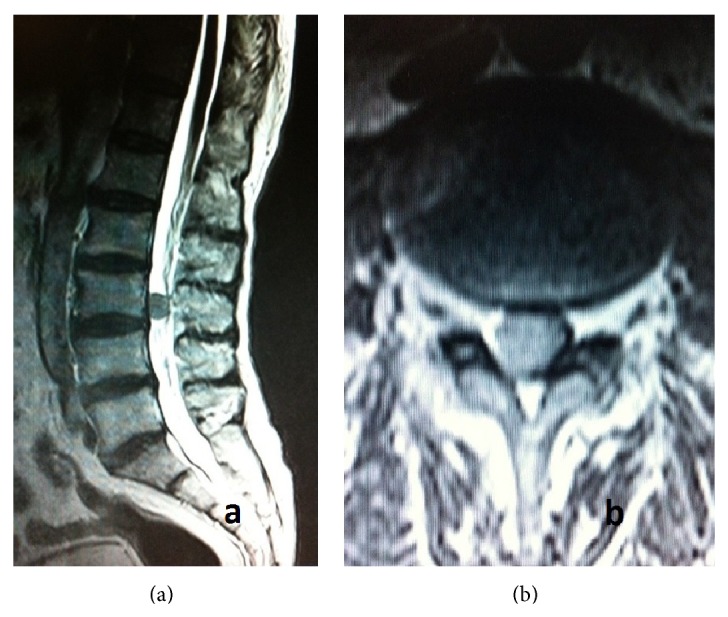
(a) Preoperative sagittal T2-weighted lumbar MRI demonstrated well-circumflanced, hypointense lesion anterior to L3 vertebrae. (b) Preoperative axial T2-weighted lumbar MRI demonstrated the well-circumferenced lesion with slightly hyperintense signal according to neural structures and hypointense signal according to CSF.
